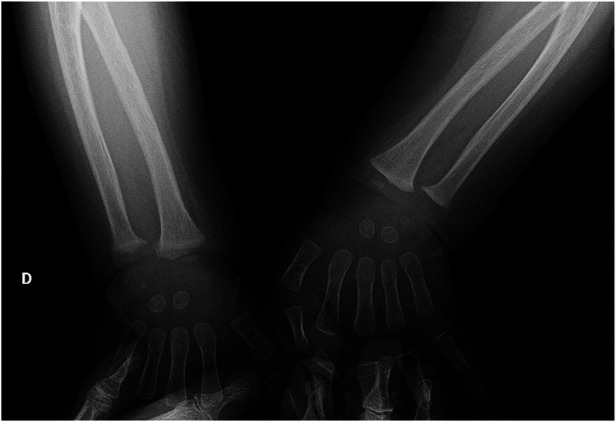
Wrist radiographs show cortical irregularities at the distal radio-ulnar metaphyseal level, prevalent in the right (D) wrist with enlargement of the growth cartilage.
